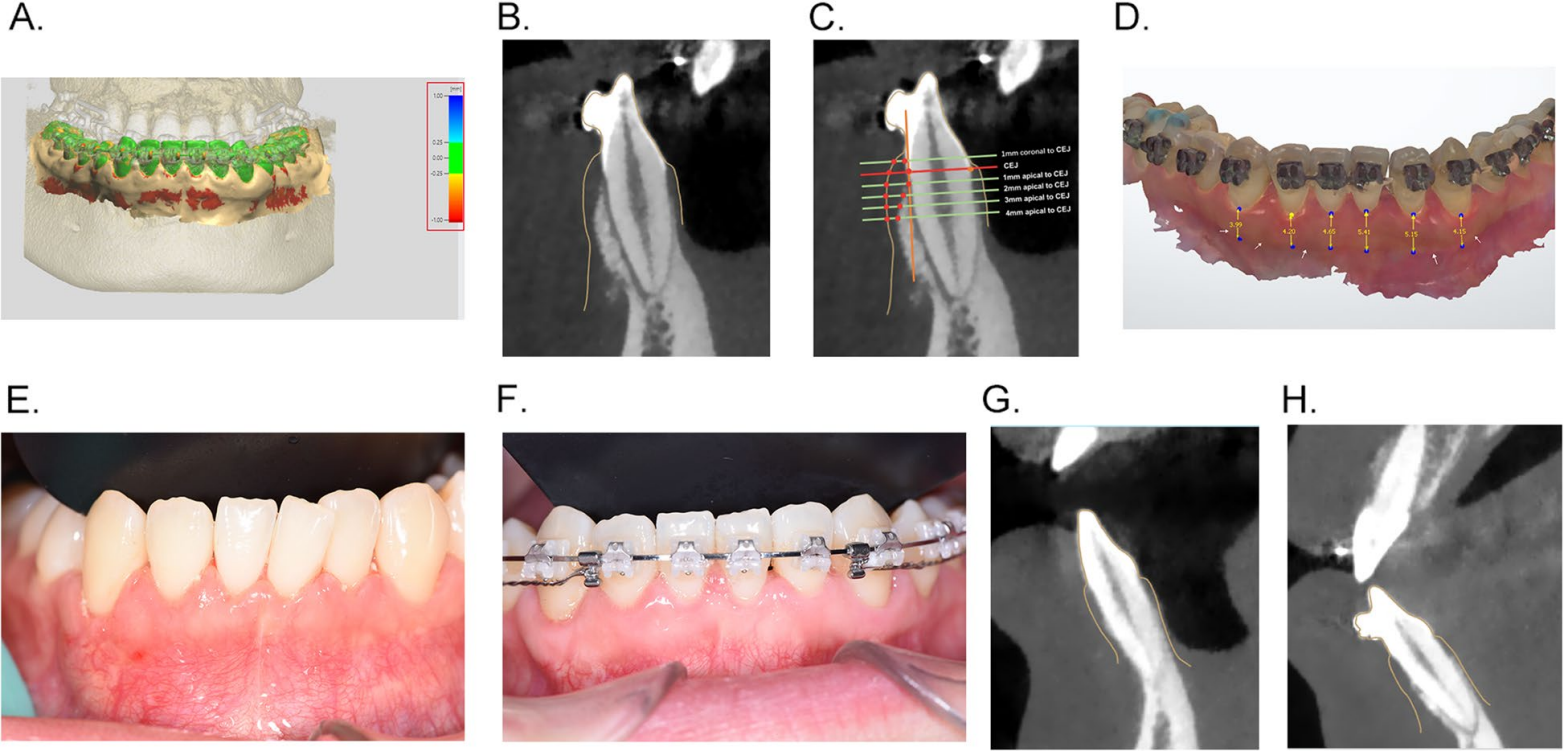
A The registration of intraoral scanning and CBCT data; B The model of the combination of intraoral scanning and CBCT data for gingival thickness measurement; C The measurement levels of gingival thickness on the combination model; D The measurement of keratinized gingiva width on the intraoral scanning model; E The representative patient intraoral photograph at T0; F The representative patient intraoral photograph at T1; G The representative patient combination model at T0; H The representative patient combination model at T1
